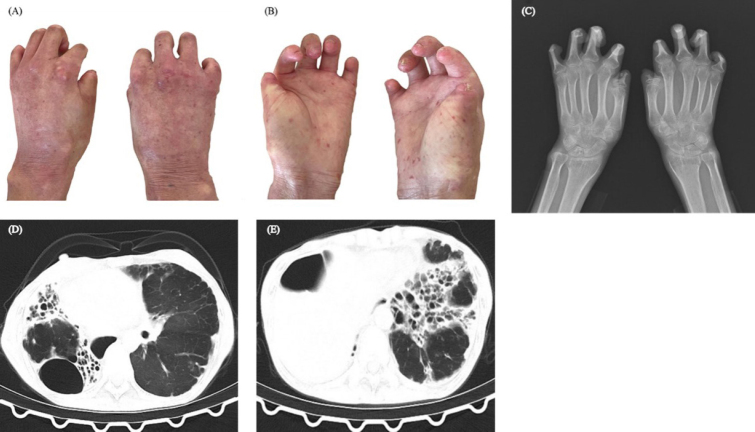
A, B: Flexion contractures of the interphalangeal joints. C: Hands X-ray presented acro-osteolysis. D, E: CT scan presented severe interstitial lung disease*.
